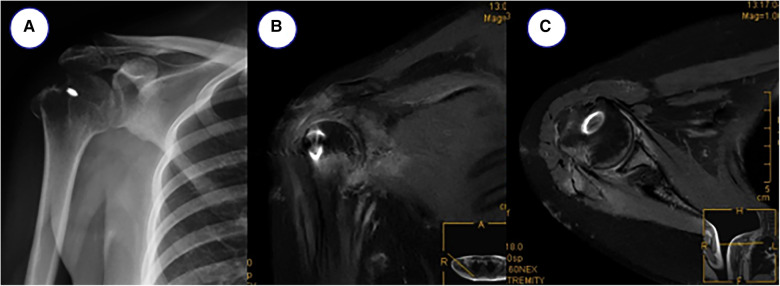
Postoperative reexamination. (A) x-ray orthopantomogram, (B,C) MRI images.
